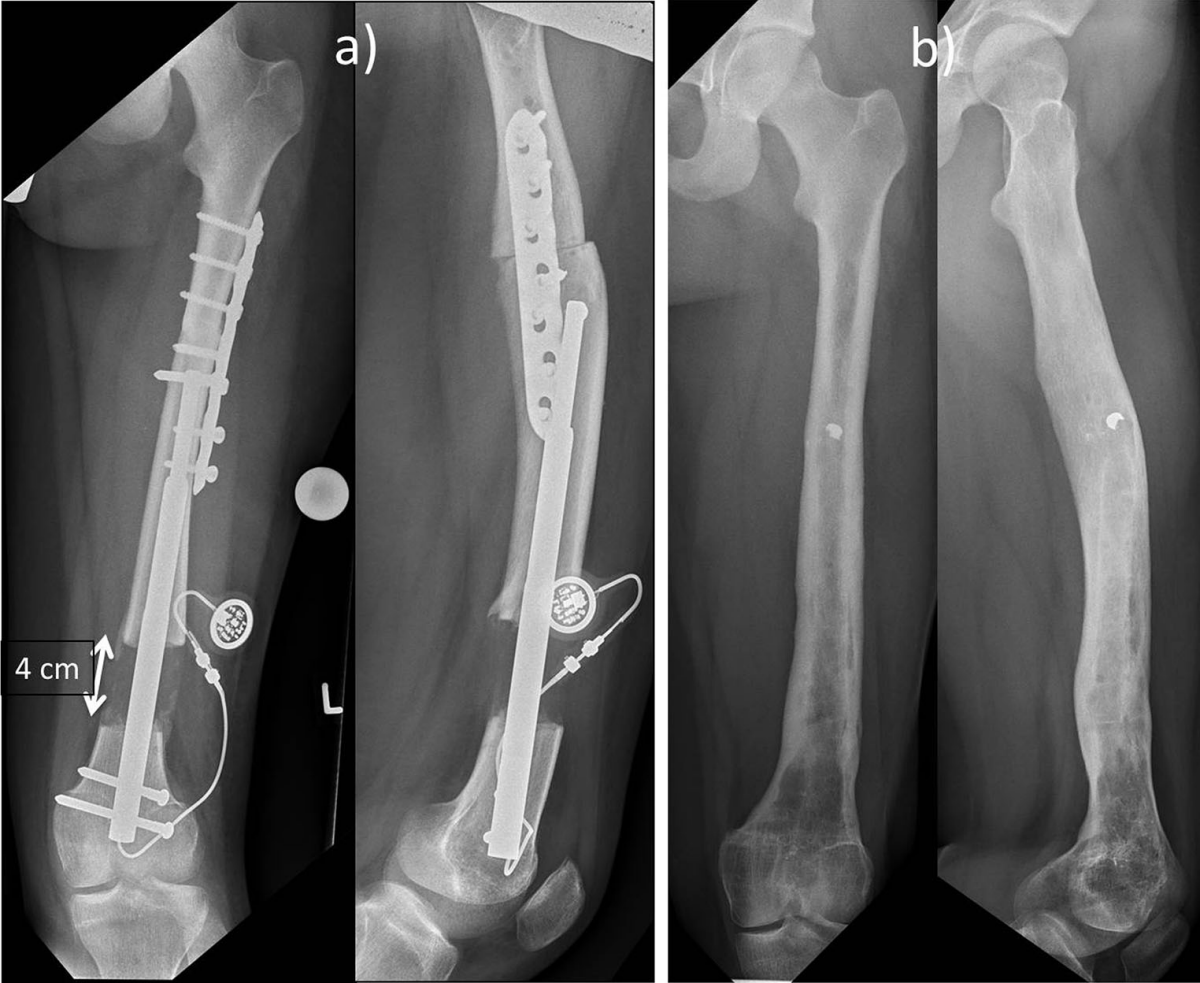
Anteroposterior and lateral X-rays after the distraction lengthening of 4 cm (a) and after removal of the metal 1.5 years later (b)
